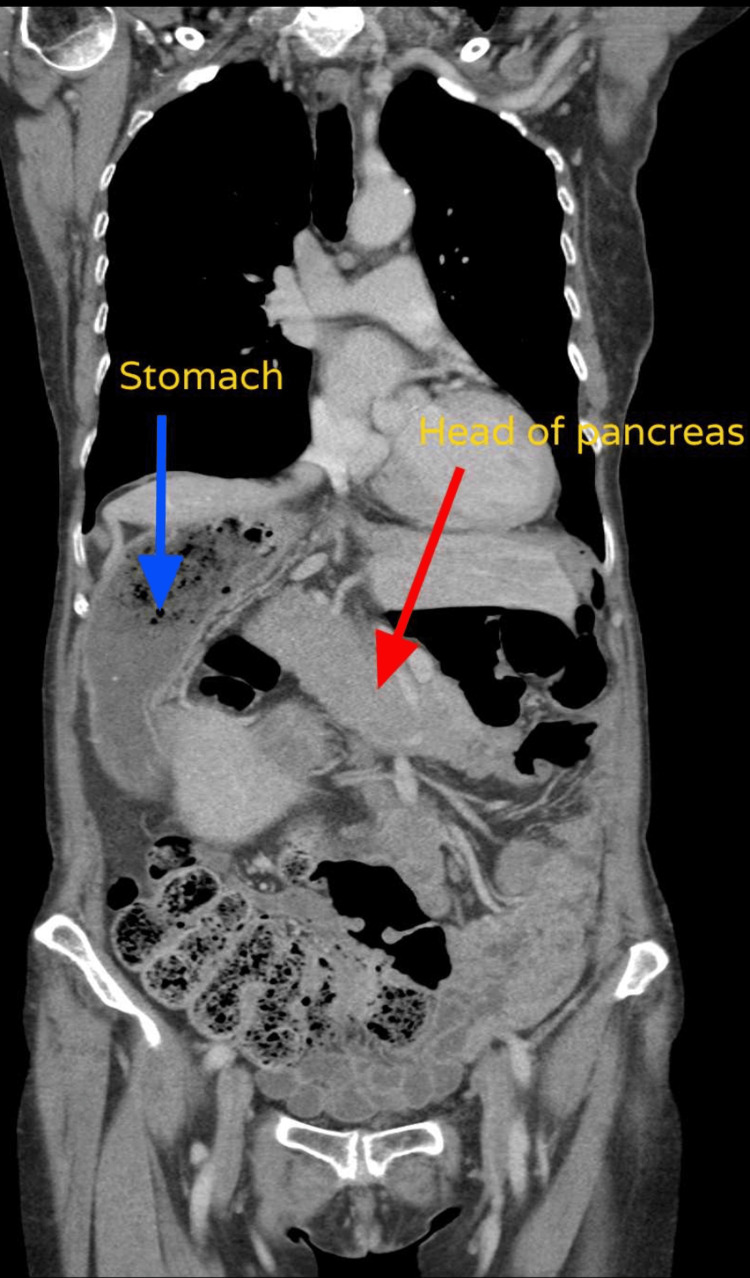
CT-TAP showing the head of the pancreas and the stomach on the right side CT-TAP: computerized tomography scan of the thorax, abdomen, and pelvis
